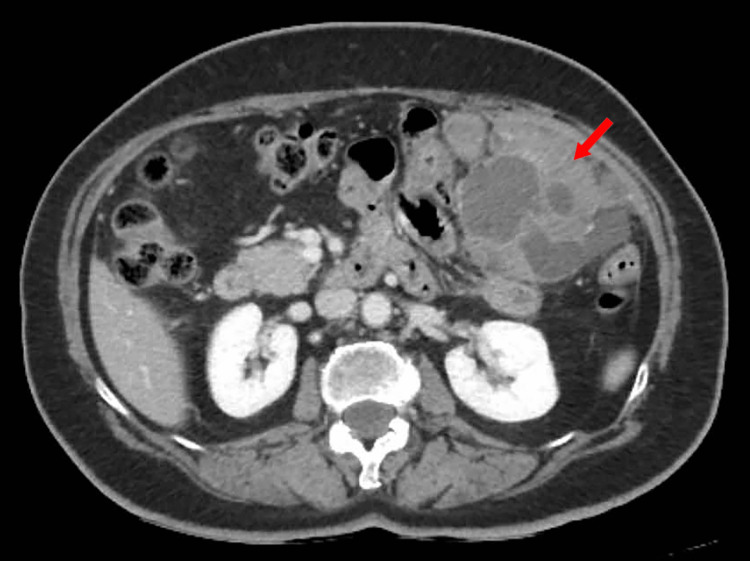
Contrast-enhanced CT demonstrating recurrent intra-abdominal tumor in 2025 Representative axial contrast-enhanced CT image shows a large intra-abdominal mass (arrow) corresponding to the lesion resected during the July 2025 multivisceral operation.
